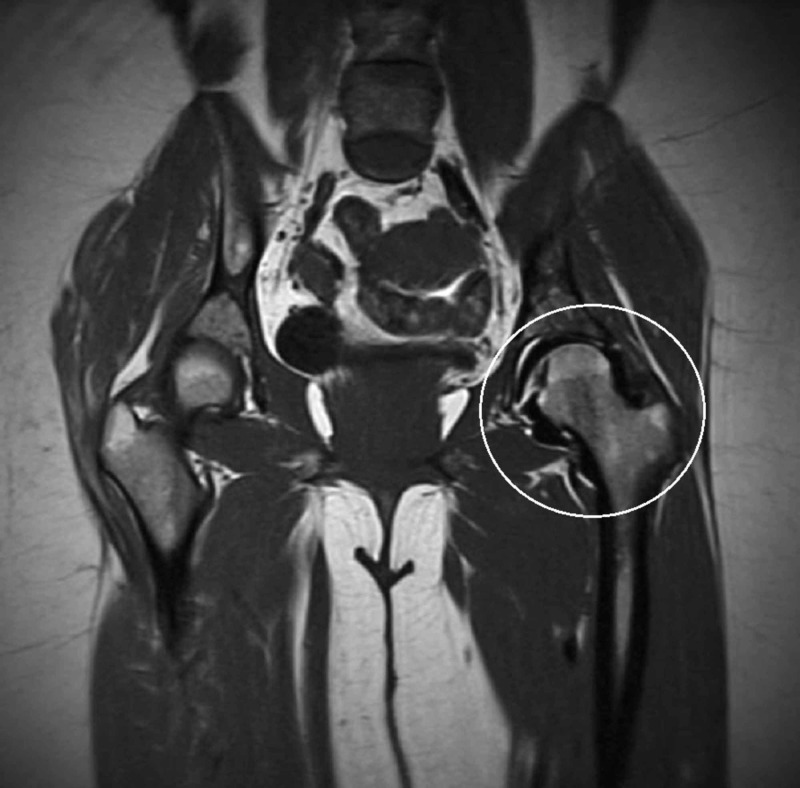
T1 weighted coronal magnetic resonance image of the pelvis The T1 weighted coronal magnetic resonance image of the left hip (white circle) exhibits a signal void surrounding the left hip and conforms to the shape of the joint capsule status post-injection for magnetic resonance arthrography.
